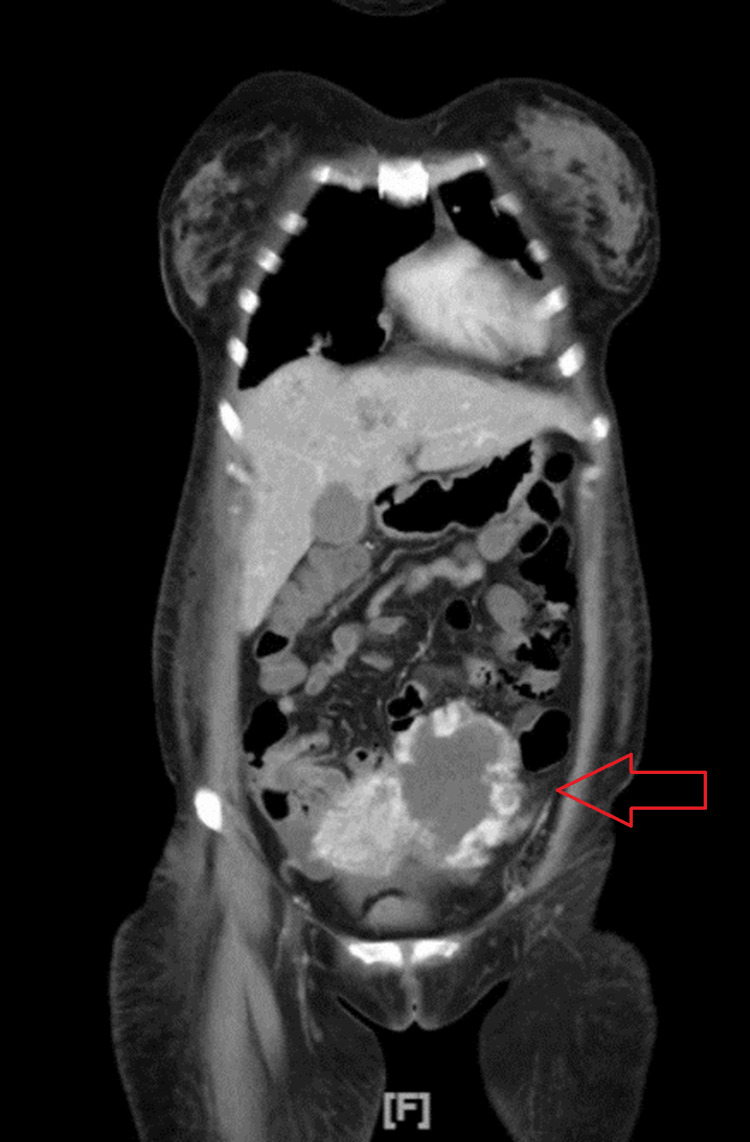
Result of abdominal CT scan showing a mass on the left lower abdomen (arrow).
